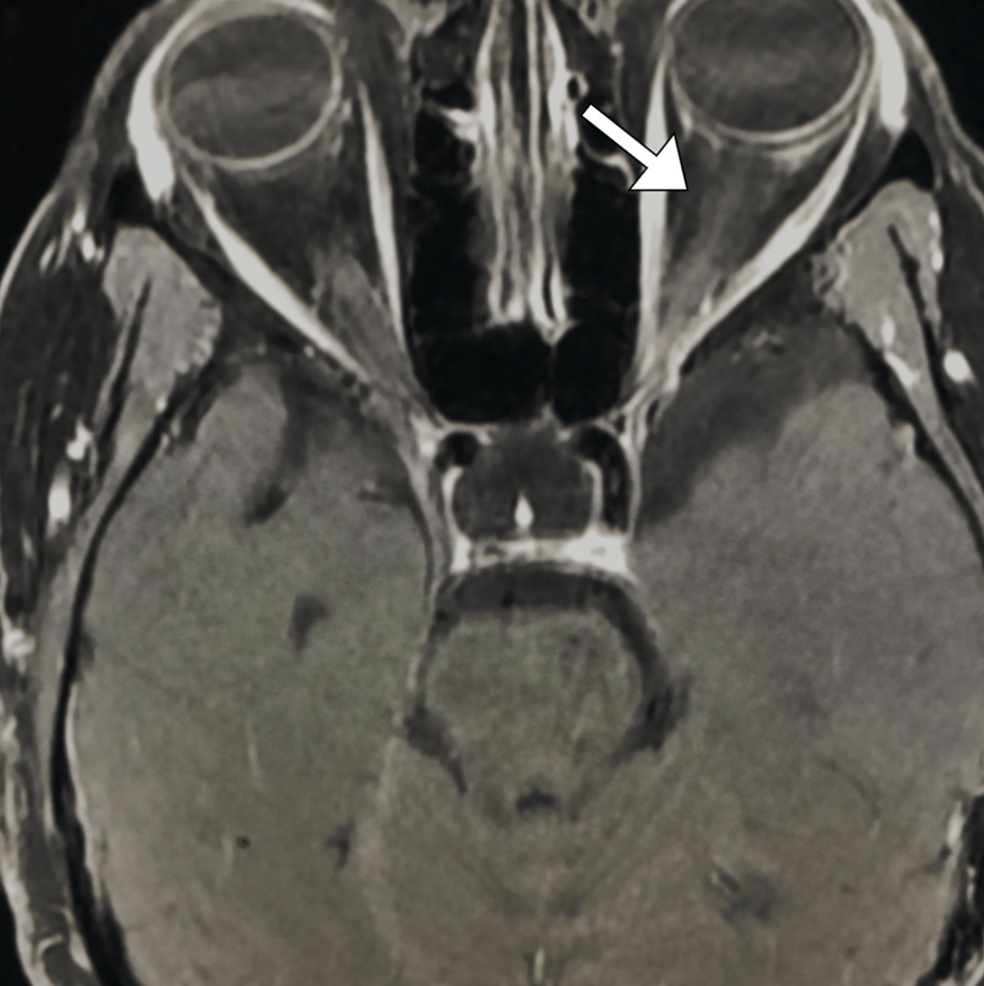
Axial MRI image with contrast demonstrating resolution of previously observed inflammatory changes in the left orbit (arrow). MRI: magnetic resonance imaging
